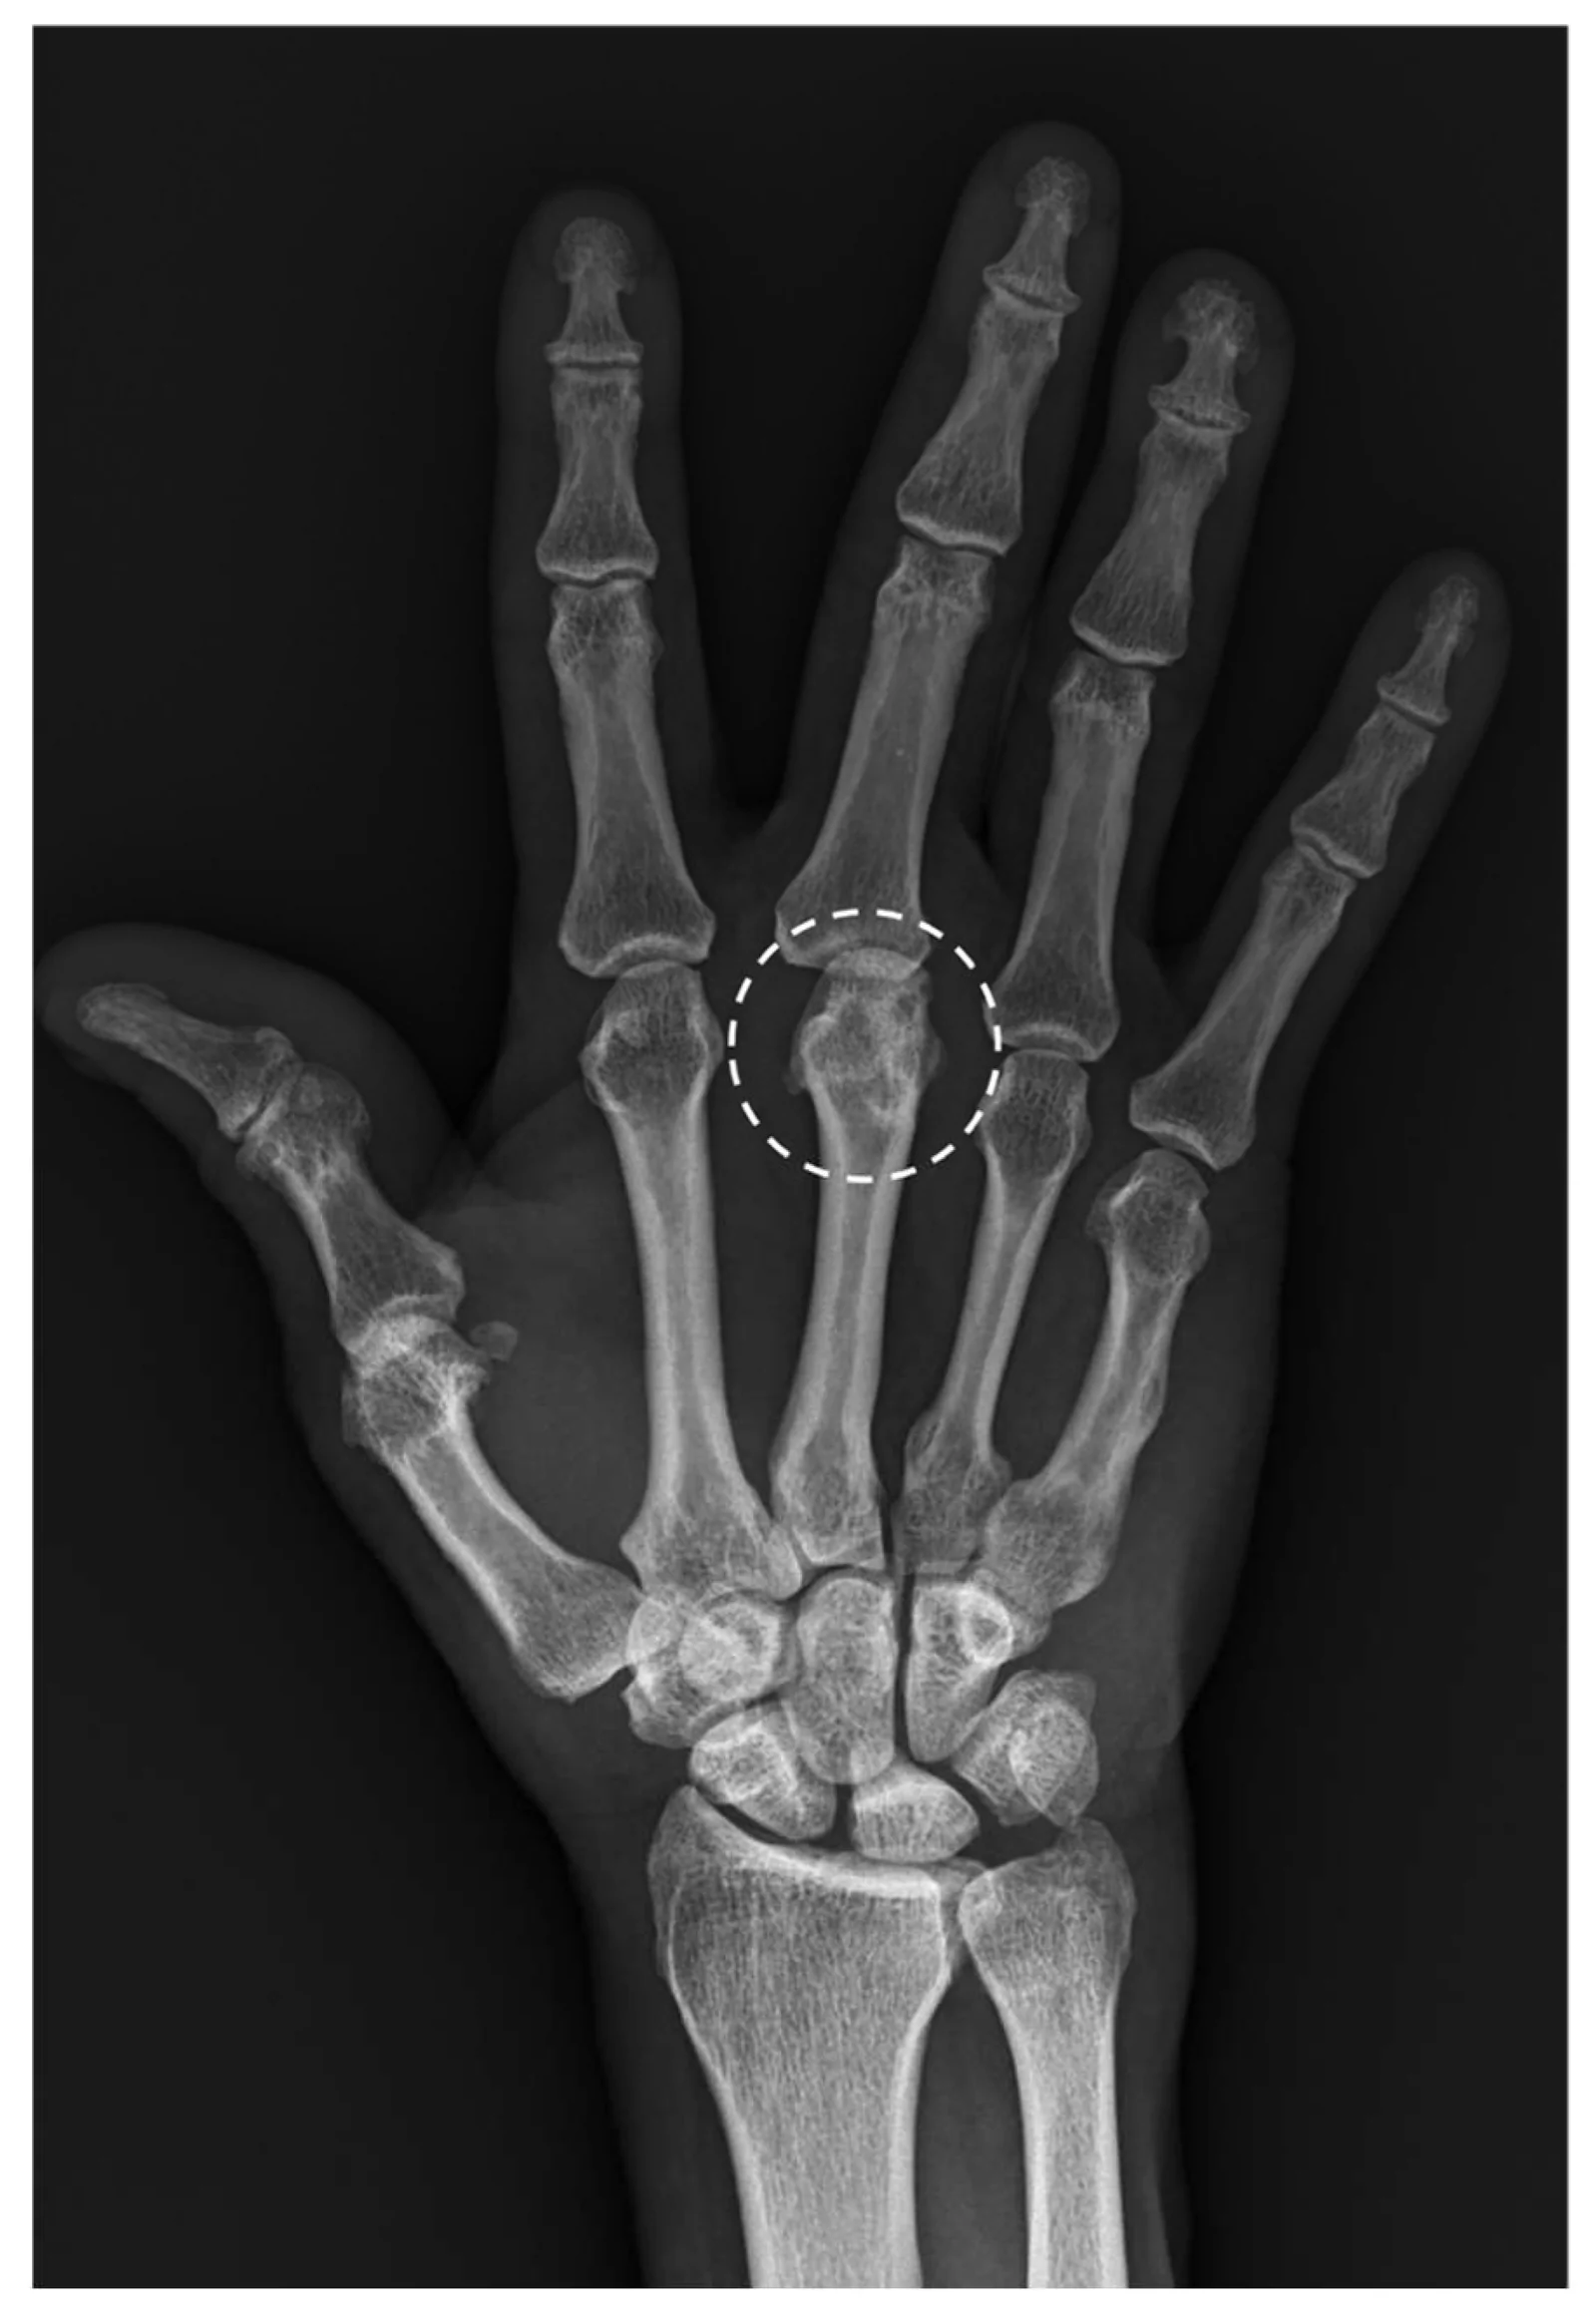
Plain radiograph of the right hand showing mixed sclerosis and subchondral lucency of the third metacarpal head (dashed circle indicates the lesion).
